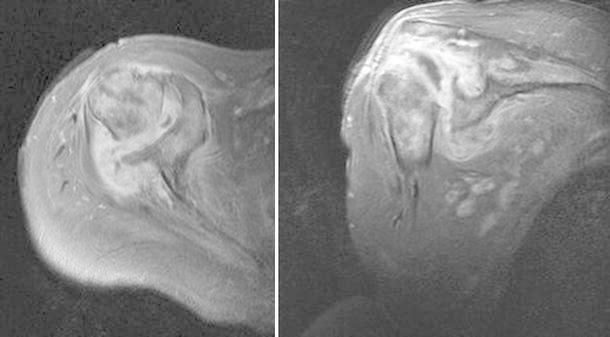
MRI imaging showing synovial inflammatory proliferation as well as contrast-enhancing joint effusion and chronic and active osteomyelitis of the humeral head and scapula. The rotator cuff muscles showed marked inflammatory changes and atrophy, and the supraspinatus muscle had lost its continuity
